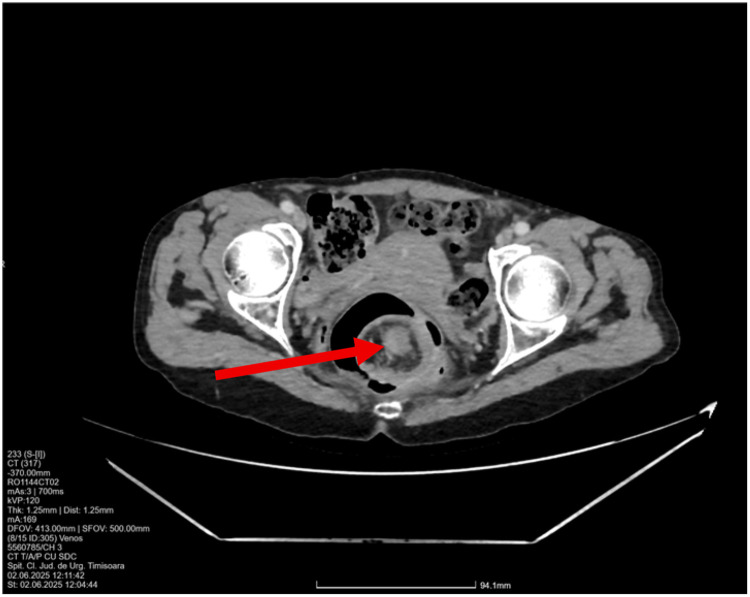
Axial contrast-enhanced CT slice through the pelvis demonstrates a bowel-within-bowel configuration within the rectal lumen, with concentric rings (“target” or “donut” sign) and invaginated mesenteric fat. The surrounding pelvic bones (iliac wings and acetabula) are visible.
